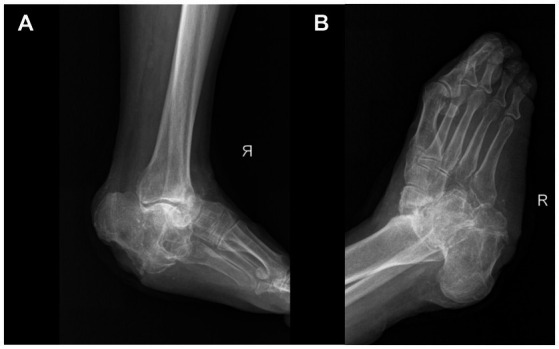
Preoperative imaging of the right foot and ankle. (A) X-ray and (B) CT scan demonstrating decreased bone density, sparse trabeculation, osteophyte formation with marginal sclerosis, and obliteration of the right calcaneocuboid joint space.
